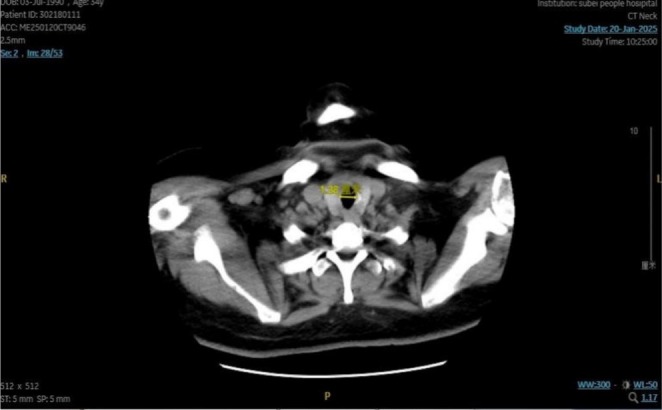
Postoperative neck CT scan.
